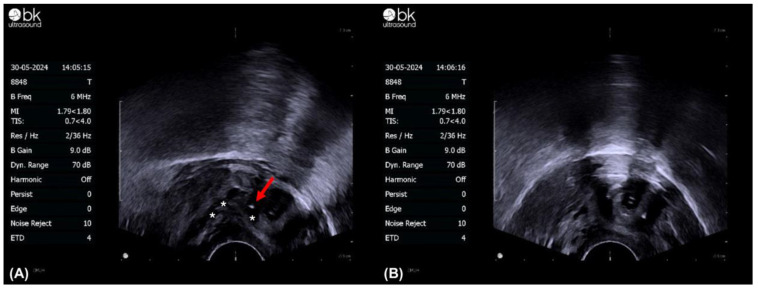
Transvaginal ultrasound-guided botulinum toxin A external urethral injection. (A,B). The external urethral sphincter’s (dark area, marked as *) precise location can be identified through transvaginal ultrasound; injection of BoNT-A; the tip of the needle is marked by a red arrow.
